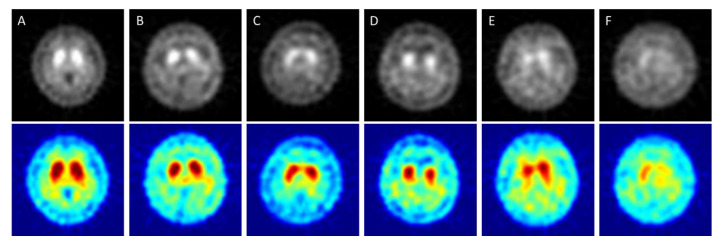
SPECT images correspond to HYS stage of PD ((A) = Healthy case, (B–F) = HYS I~V). The Upper row is original grayscale images. The lower images have pseudocolor mapping.
